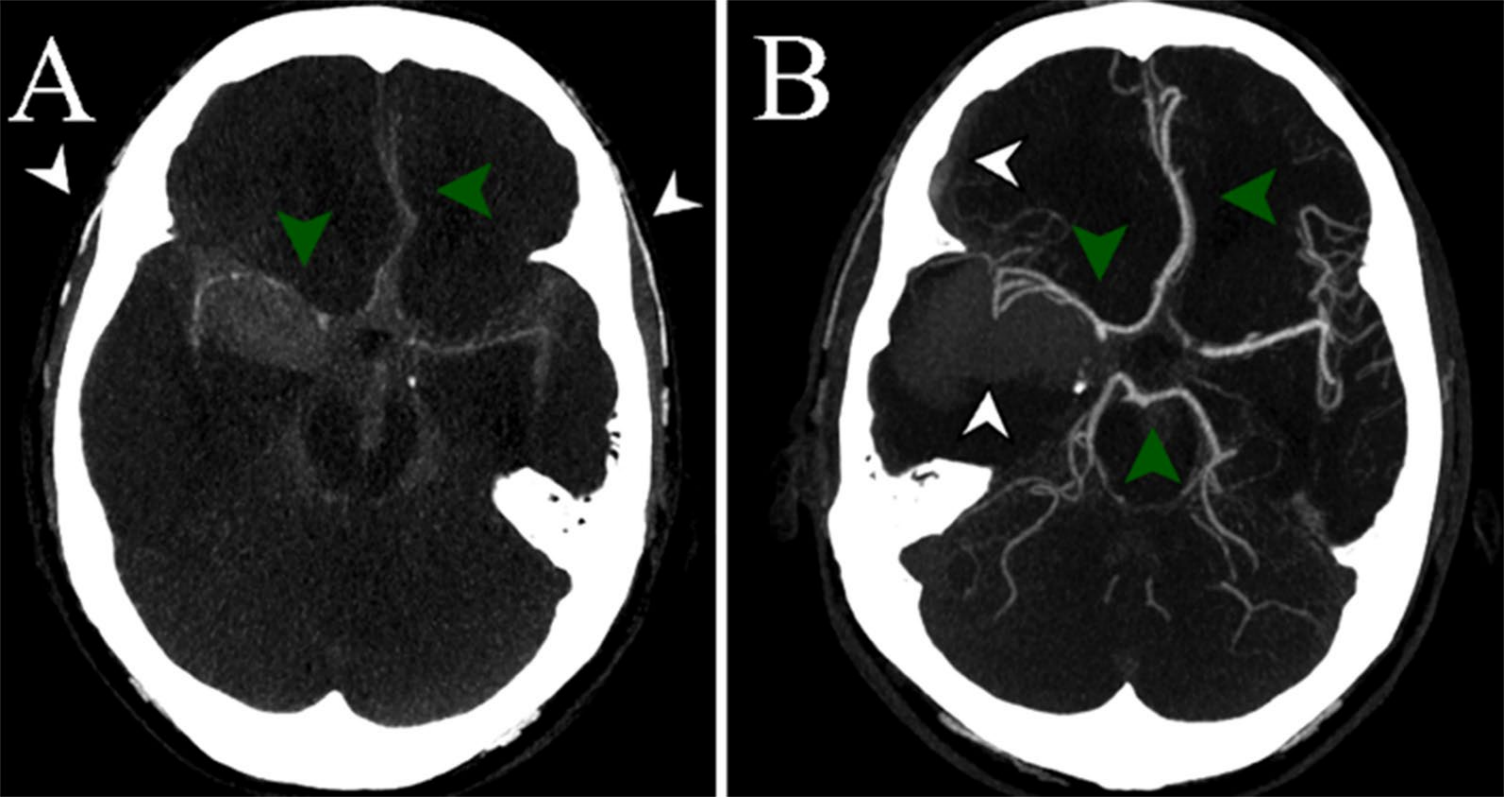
Computed tomography angiography (CTA) scans. At the time of aneurysm rupture and intracranial circulatory arrest (a), anterior and middle cerebral arteries filled vaguely (a; green arrowheads) whereas extracranial temporal arteries were clearly viewed (a; white arrowheads). After regaining intracranial circulation (b), anterior, middle and posterior cerebral arteries were filling normally again (b; green arrowheads). CTA showed also signs of a rebleed, such as a grown intracerebral hematoma and a new right acute subdural hematoma (b; white arrowheads). Computed tomography scan confirmed the rebleed (images not shown) (Color figure online)
